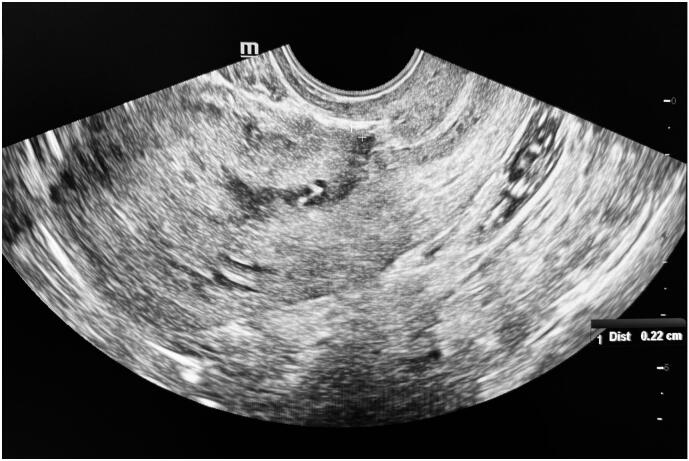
Transvaginal ultrasound revealing the isthmocele. Pelvic ultrasound shows a normal-sized uterus with a thin endometrium and a triangular, anechoic defect in the anterior uterine wall at the site of the previous cesarean section. The residual myometrial thickness at the thinnest point measures 2.2 mm (white calipers).
